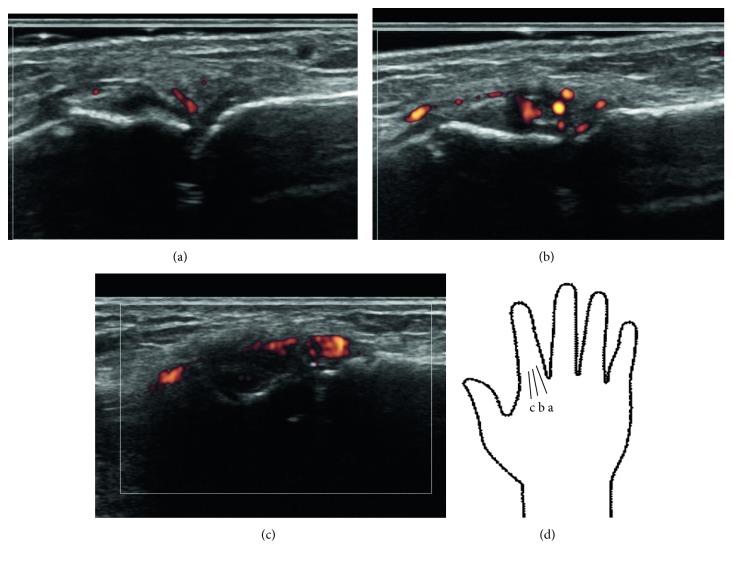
Ultrasonography of the metacarpophalangeal joint of the right index finger. No remarkable findings were seen on a midline echo (a), but synovial fluid and power Doppler signals were noted on the radial-shifted view (b) and (c).
